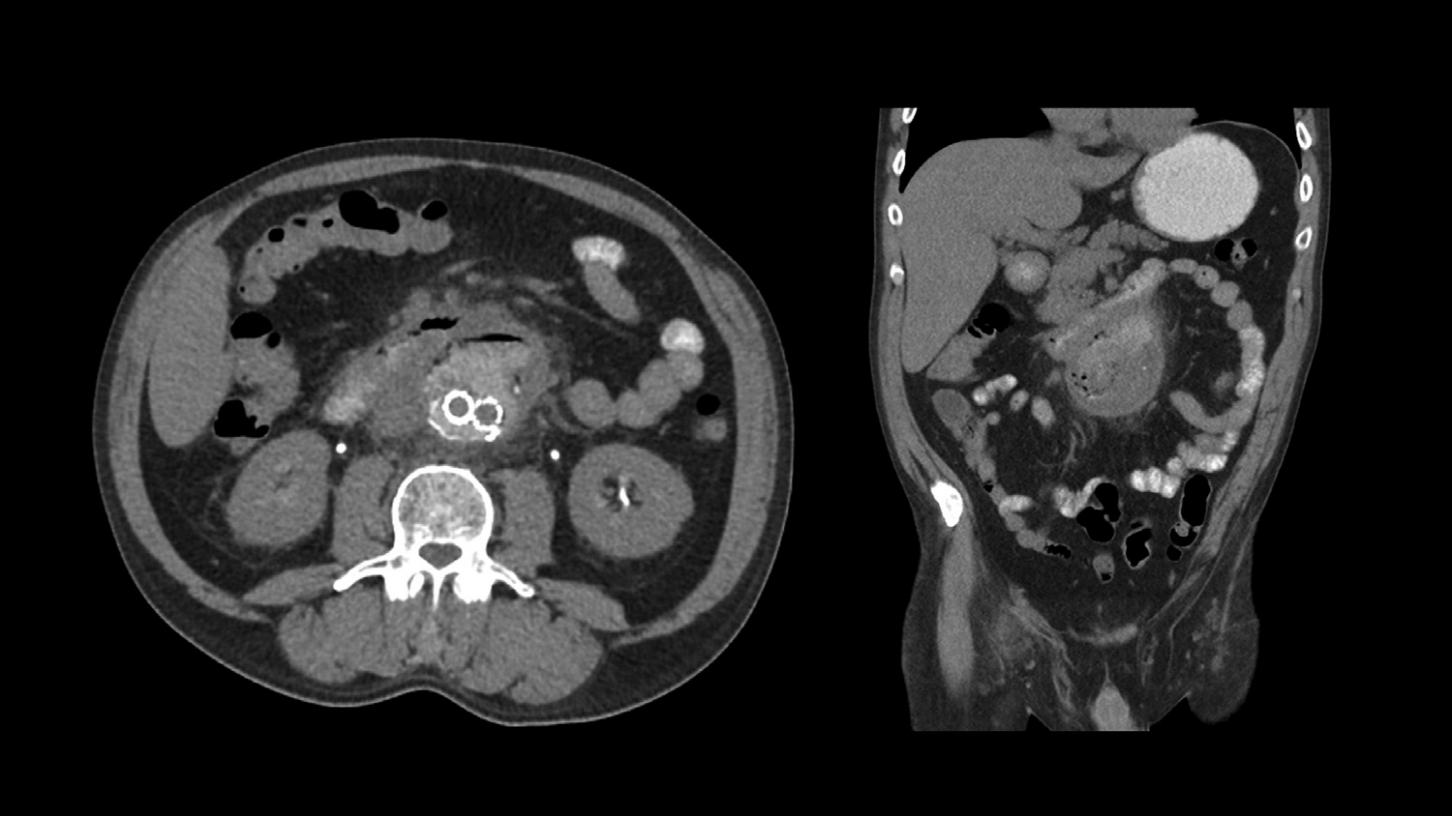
Prior to transfer, CT abdomen and pelvis with enteric contrast was performed which showed enteric contrast into the excluded aneurysmal sac, again confirming known aortoenteric fistula.
